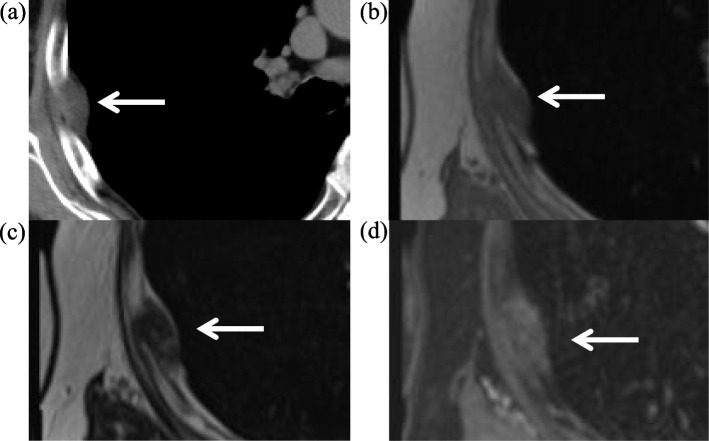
a Preoperative computed tomography. A soft-tissue mass measuring 3.1 × 1.5 cm (arrow) is seen in the right fifth intercostal space without bone involvement. b Preoperative T1-weighted MRI. The tumor (arrow) displays intermediate intensity on T1-weighted imaging. c Preoperative T2-weighted MRI. The tumor (arrow) displays low intensity on T2-weighted imaging. d Preoperative enhanced MRI. The tumor (arrow) displays heterogeneous enhancement
